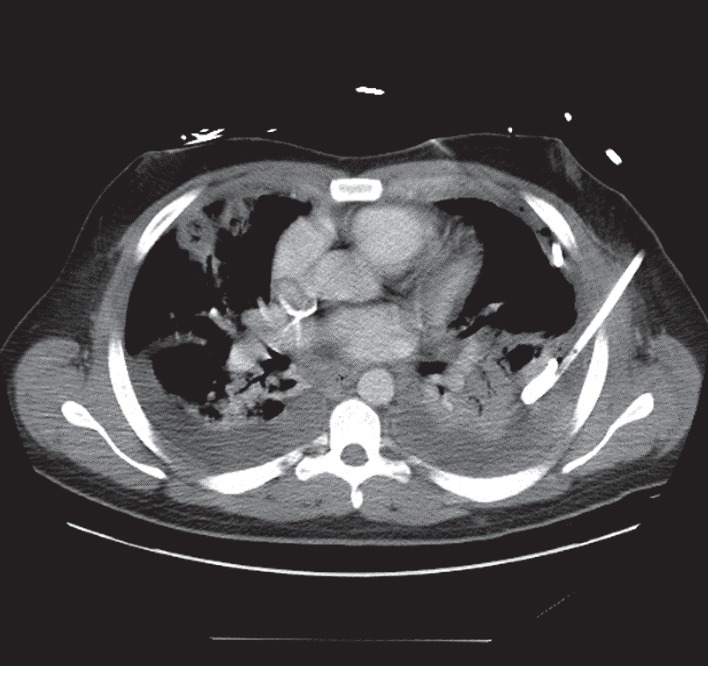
Horizontal section of chest computed tomography (CT) with contrast showing left-sided, multiloculated pleural effusion, status postplacement of pigtail catheter. Multifocal areas of necrotizing pneumonia and multiple pulmonary abscesses with smaller right-sided pleural effusion are also shown.
